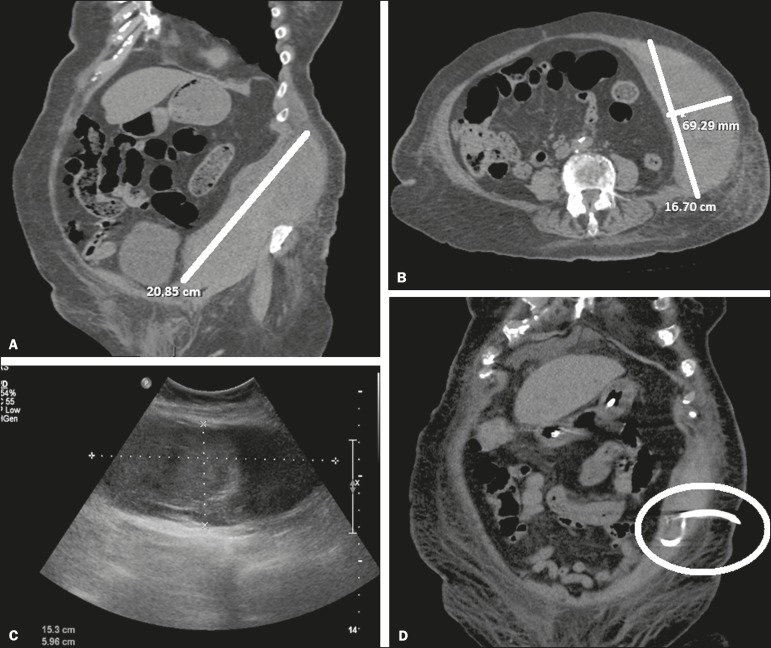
A,B: Computed tomography showing hematoma of the abdominal
